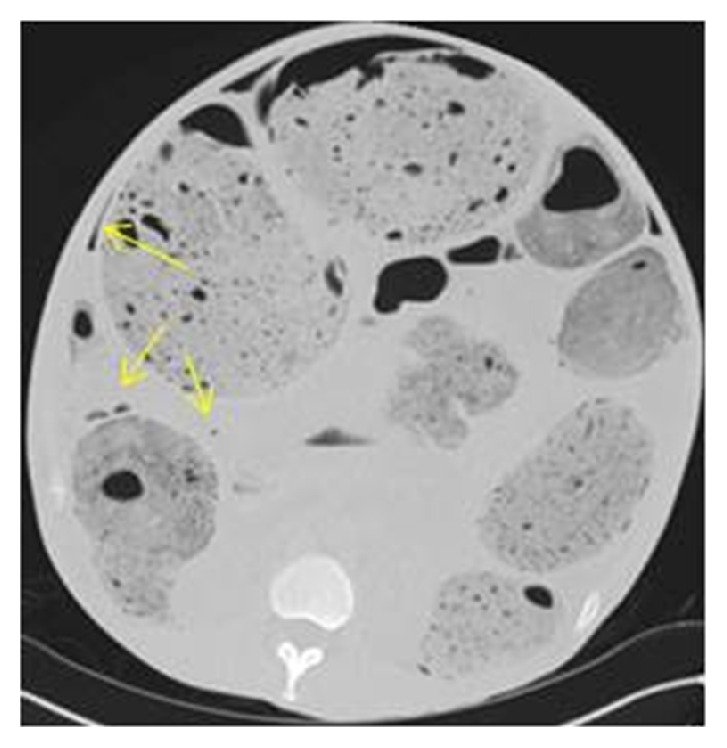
Axial CT images on lung windows confirming small pockets of free gas adjacent to the perforation site.
